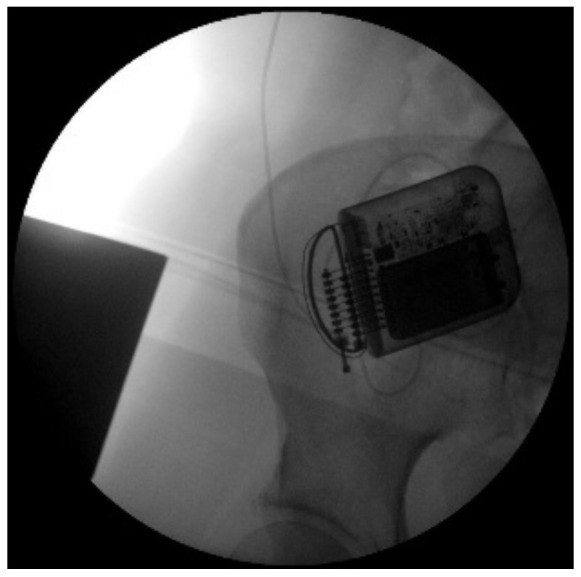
X-ray image of the neurostimulator in right iliac fossa.
